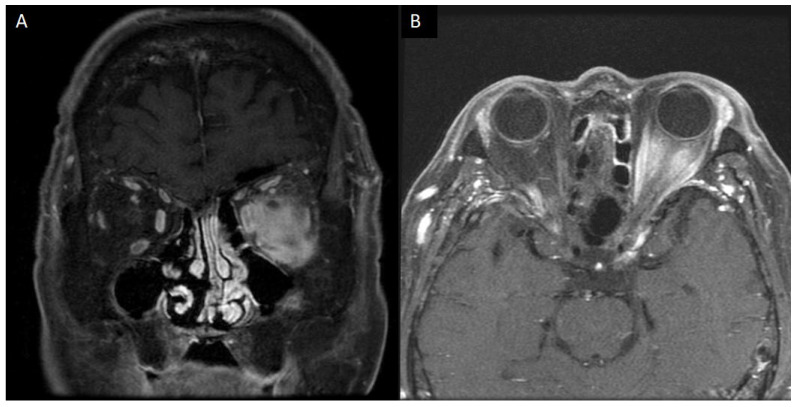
After treatment, the orbital MRI revealed a remarkable resolution of inflammation (A,B).
